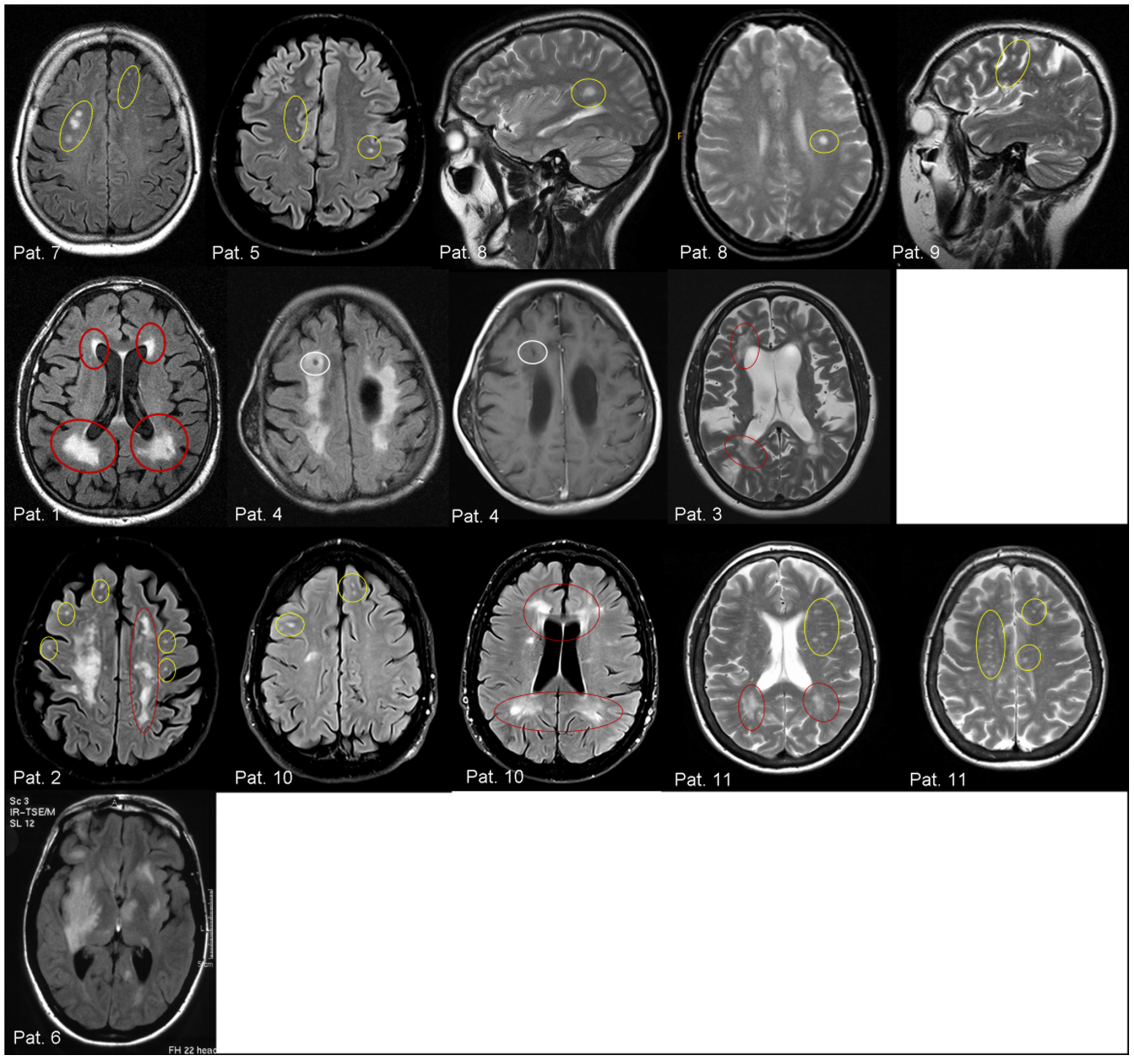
MR images (T2-weighted and FLAIR sequences) of 11 Fabry patients misdiagnosed with multiple sclerosis. *Upper row:* Patients 7, 5, 8 and 9 showed more punctuate and subcortical white matter lesions (yellow circles). *Second row:* White matter lesions in patients 1, 4, and 3 had a rather confluent, periventricular pattern (red circles). This pattern was associated with “black holes” (white circles) in T1-weighted images as a surrogate of severe demyelination and axonal injury. *Third row:* Patients 2, 10, and 11 revealed both disseminated and confluent, periventricular patterns. *Fourth row:* Only patient 6 showed an uncommon image with a severe, asymmetrical involvement of cortical, subcortical, and basal ganglia tissues.
